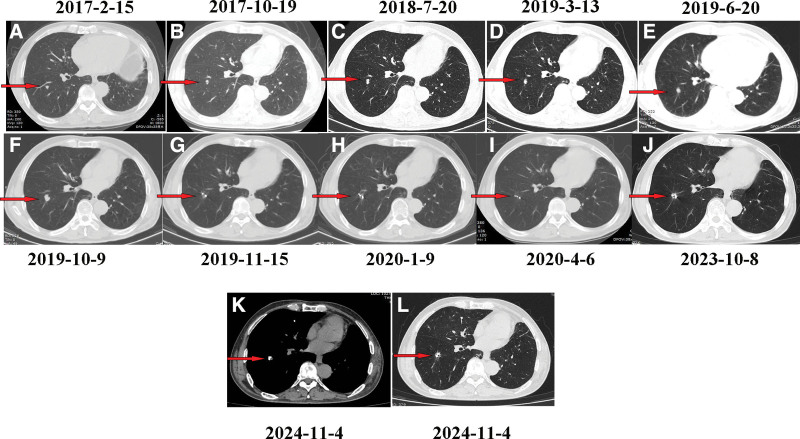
Lung CT images. (A) February 15, 2017: A new metastatic lesion (arrow) in the right lower lobe detected after 3 cycles of paclitaxel plus cisplatin chemotherapy. (B) October 19, 2017: The metastatic lesion (arrow) remains stable in size after 6 cycles of pemetrexed plus bevacizumab. (C) July 20, 2018: Continued stability of the lesion (arrow) was observed after 12 months of bevacizumab maintenance therapy. (D) March 2019: The lesion (arrow) measuring 12 mm × 10 mm. (E) June 20, 2019: The lesion (arrow) remains stable (12 × 10 mm) after 23 months of bevacizumab treatment, showing no significant change from the previous scans. (F) October 9, 2019: Oligoprogressive disease is noted, with enlargement of the lesion (arrow) to 15.2 mm × 14.6 mm after 27 months of bevacizumab, before ^¹²⁵^I brachytherapy. (G) November 15, 2019: 1 month after ^¹²⁵^I implantation, no recurrence is observed. (H) January 9, 2020: 3 months postimplantation, no recurrence was observed. (I) April 6, 2020: At 6 months postimplantation, there was no evidence of recurrence. (J) October 8, 2023: No local recurrence was evident 48 months after brachytherapy. (K) November 4, 2024: Mediastinal window view 61 months postimplantation shows no recurrence. (L) November 4, 2024: Lung window view at 61 months postimplantation confirms no recurrence. CT = computed tomography.
